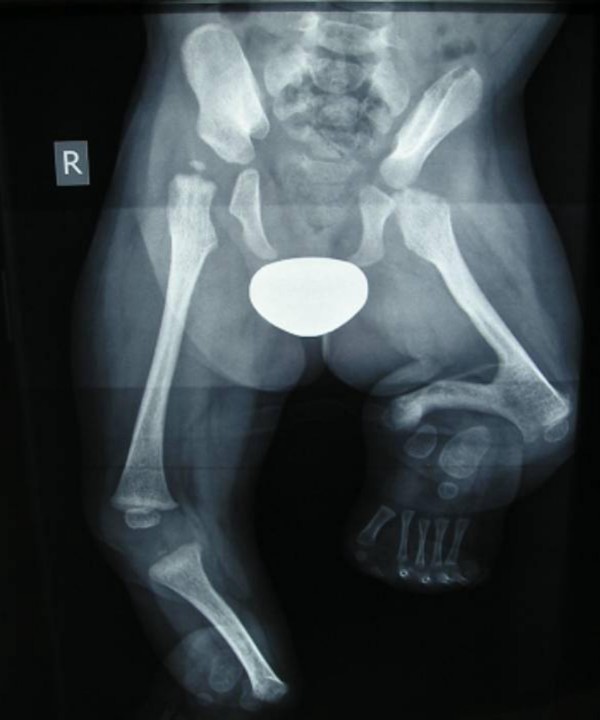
Radiographic examination of the pelvis and the lower limbs showed hypoplastic iliac bones, a hypoplastic left femur with subsequent development of femoral-tibial synostosis. Fixed flexion deformities of the knees was apparent, but with maximal intensity over the left knee. Bilateral fibular aplasia, the ankles showed defective ossification associated with hypoplasia of the distal phalange.
